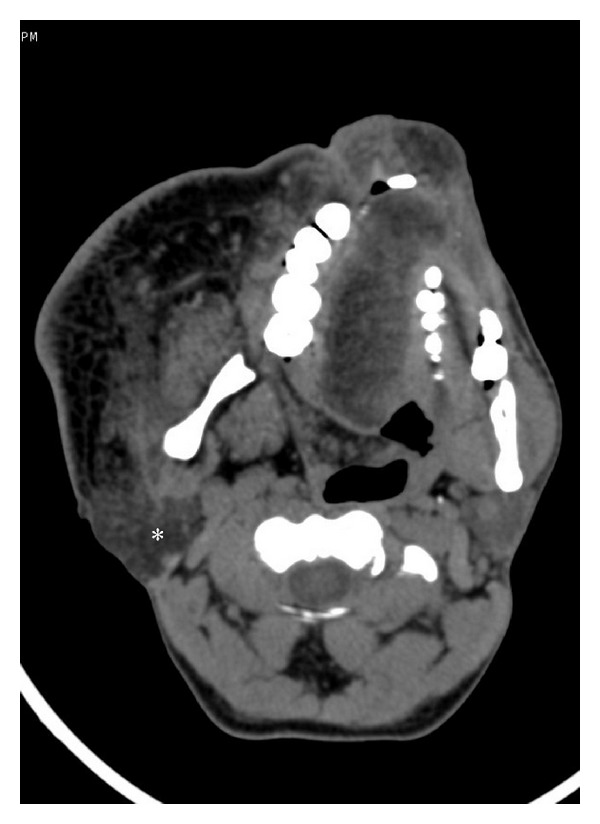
Axial CT scan showing lipomatous enlargement of the soft tissue on right side of face including buccal region, lips, tongue, soft palate, and right parotid gland (asterix).
